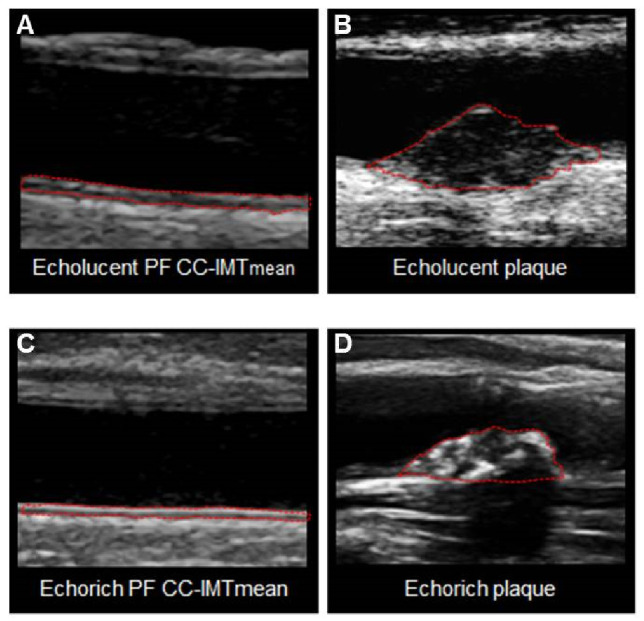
Examples of lesions with echolucent (A,B) and echo-rich (C,D) features of two types of carotid ultrasonographic images. Panels on the left show the echolucency features detected on the common carotid IMT, measured in plaque free areas (echolucency of PF CC-IMTmean). Panels on the right show the same features detected on a carotid plaque (echolucency of plaque).
